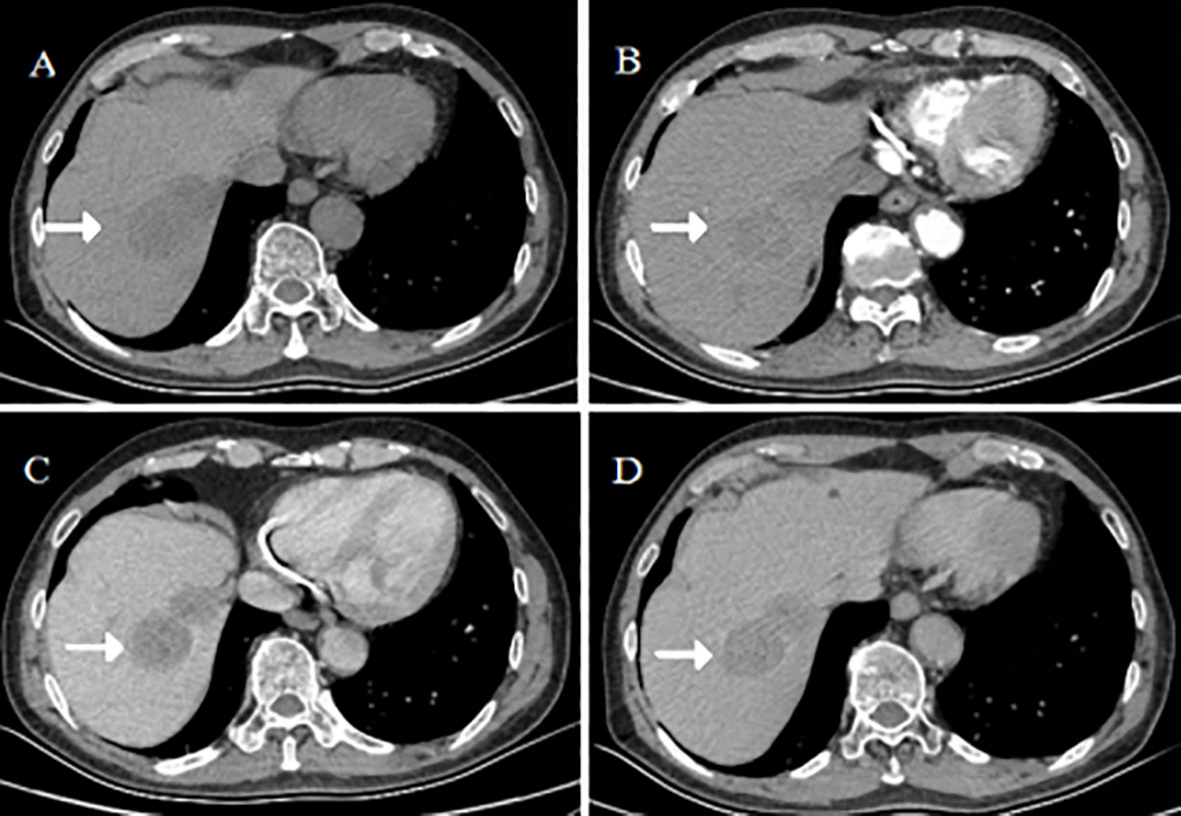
Surveillance CT detecting liver metastases (February 2021). Axial post-contrast CT images in (A) arterial and (B) portal venous phases reveal new, rim-enhancing metastatic lesions in the right hepatic lobe (white arrows). The largest metastasis in segment VII is shown in (C) axial and (D) coronal planes.
